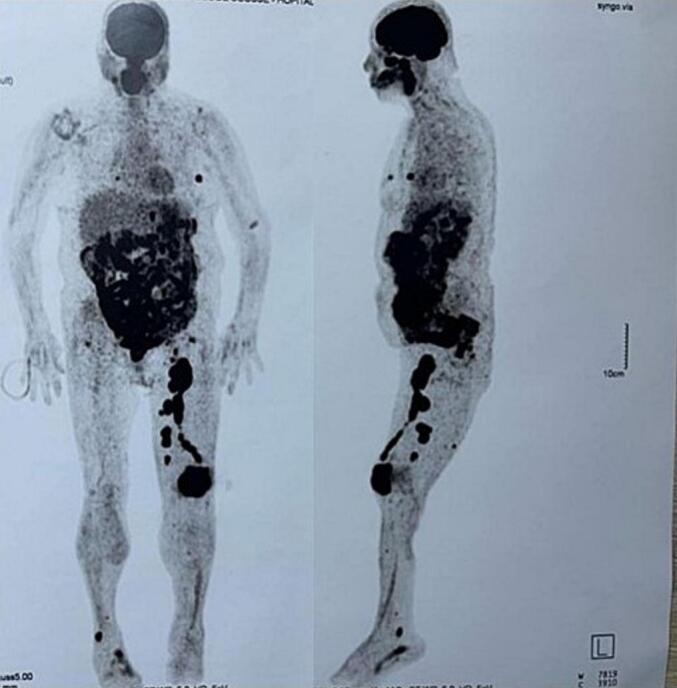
Follow-up PET/CT demonstrating systemic progression at 6 months:* Fused FDG PET/CT images showing multiple new sites of intense hypermetabolism including widespread lymphadenopathy bilateral adrenal glands, multiple osseous lesions with persistent marked fixation in the right patella, mammary, and subcutaneous involvement, indicating extensive disease progression.
